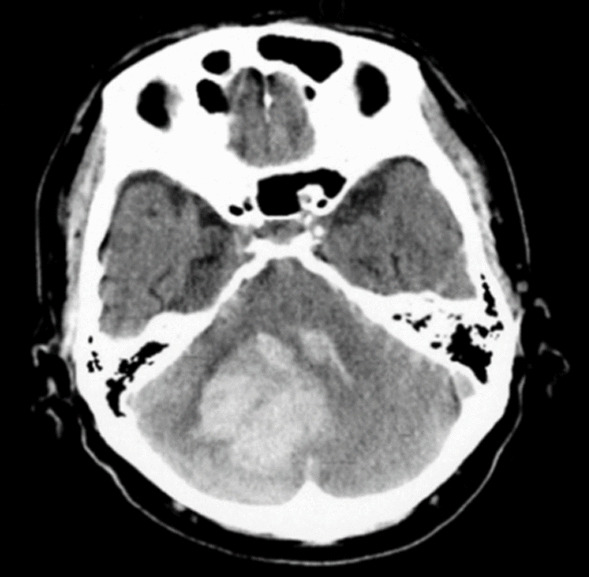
CT image of the head at onset. Hemorrhage in the right cerebellar peduncle and hemisphere (hemorrhage volume 37 mL). *CT* computed tomography
